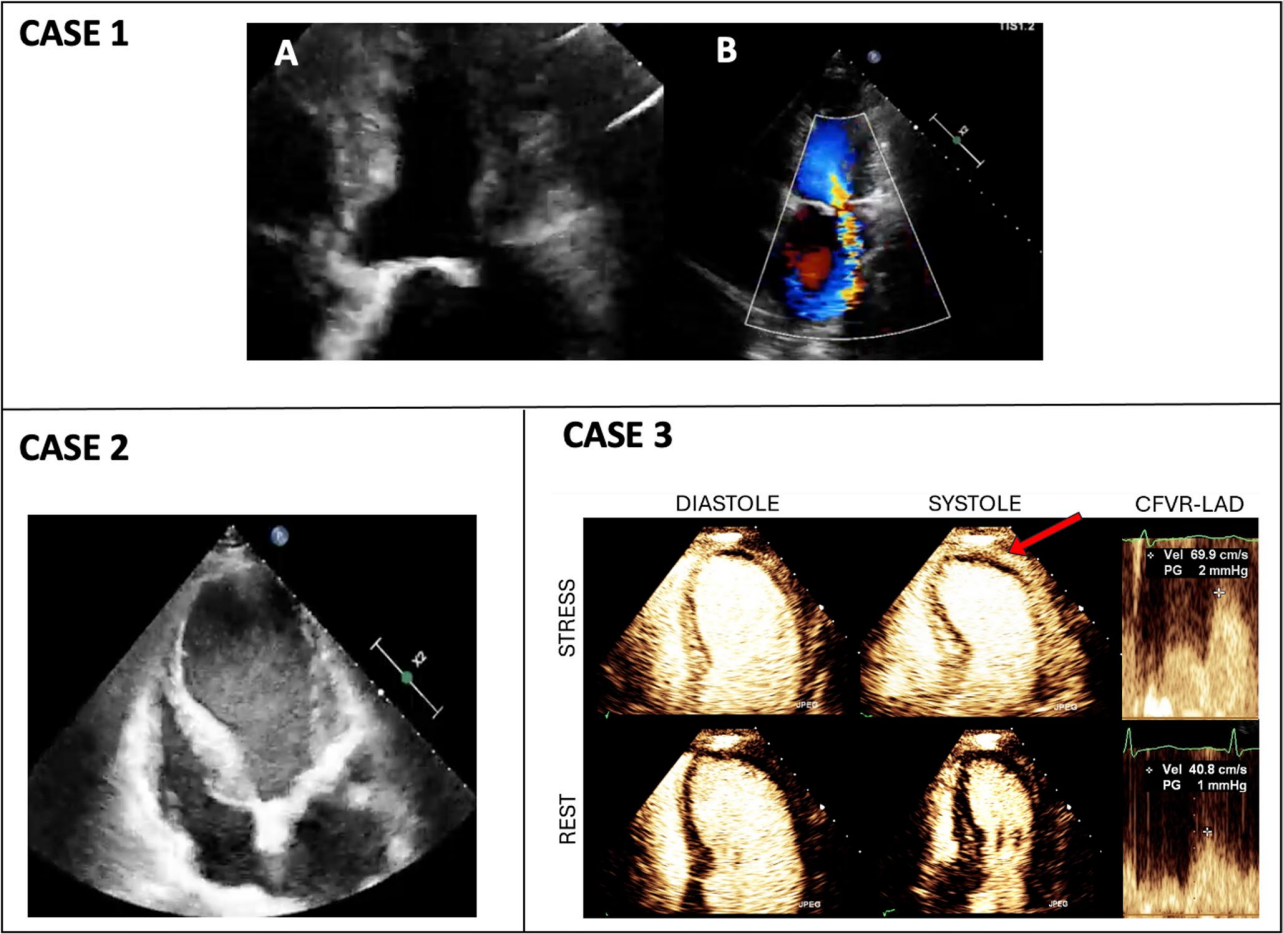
Echocardiographic evaluation in ACS. *Case 1*: Late-presenter STEMI with thrombotic occlusion of the first diagonal and intermediate branches, causing partial rupture of the antero-lateral papillary muscle and severe mitral regurgitation (1A, 1B). *Case 2*: Aneurysmal LV apex following anterior STEMI with 'smoke' effect. *Case 3*: Suspected UA patient underwent stress MCE post-ED discharge, showing normal wall motion at rest. After dipyridamole infusion, systolic akinesia of the LV apex (arrow) with perfusion defect and reduced CFVR-LAD of 1.7. Abbreviations:MCE, myocardial contrast echocardiography; ED, emergency department; LV, left ventricular; CFVR, coronary flow velocity reserve, and as in Figure 1
